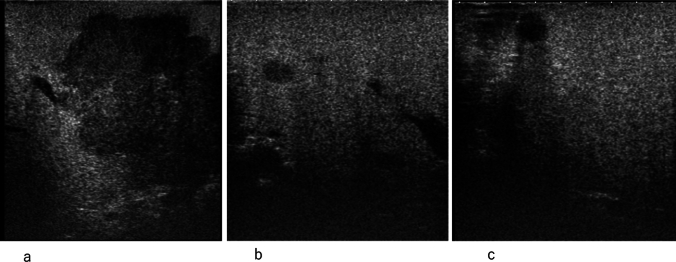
Metastatic liver tumors. a, b, c, Sonazoid contrast-enhanced ultrasound enables the detection of small malignant liver lesions
